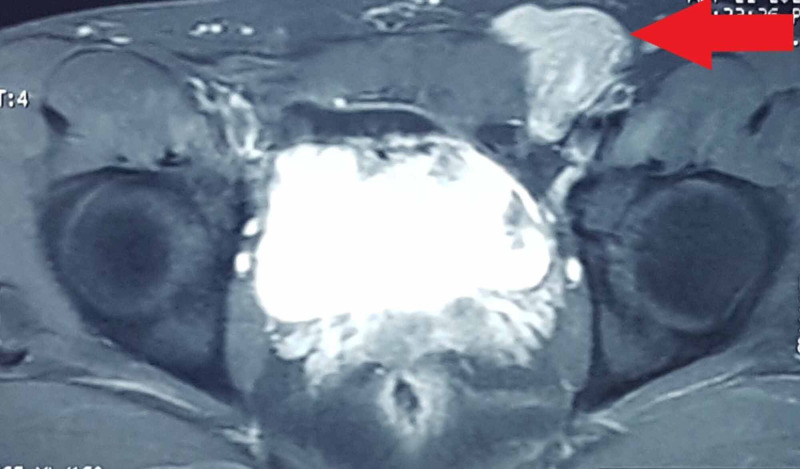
MRI T1-weighted post-contrast showing enhancement of left spermatic cord.
